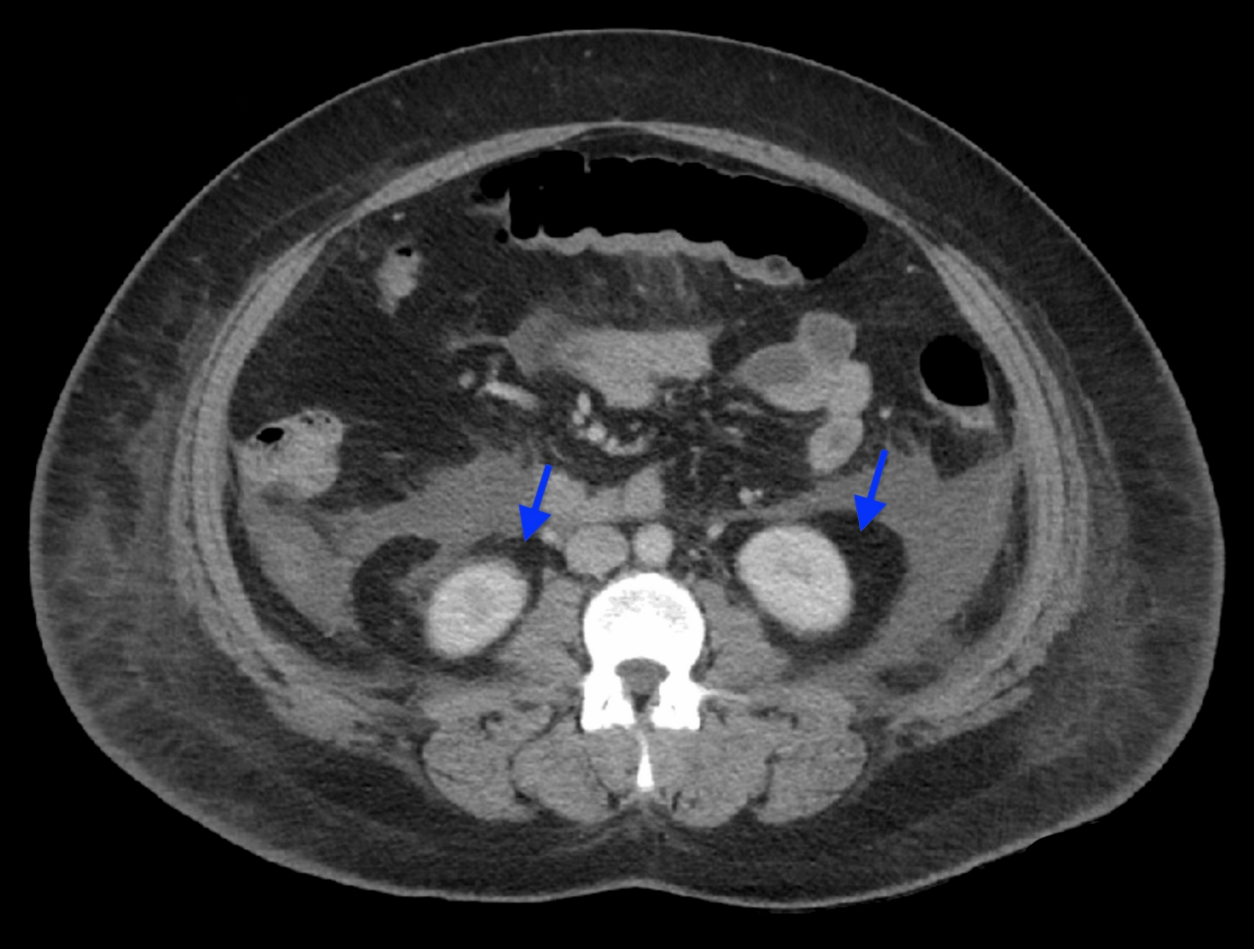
Computed tomography scan showing acute pancreatitis associated with extensive extra-pancreatic fluid in the right and left anterior pararenal spaces (blue arrows).
